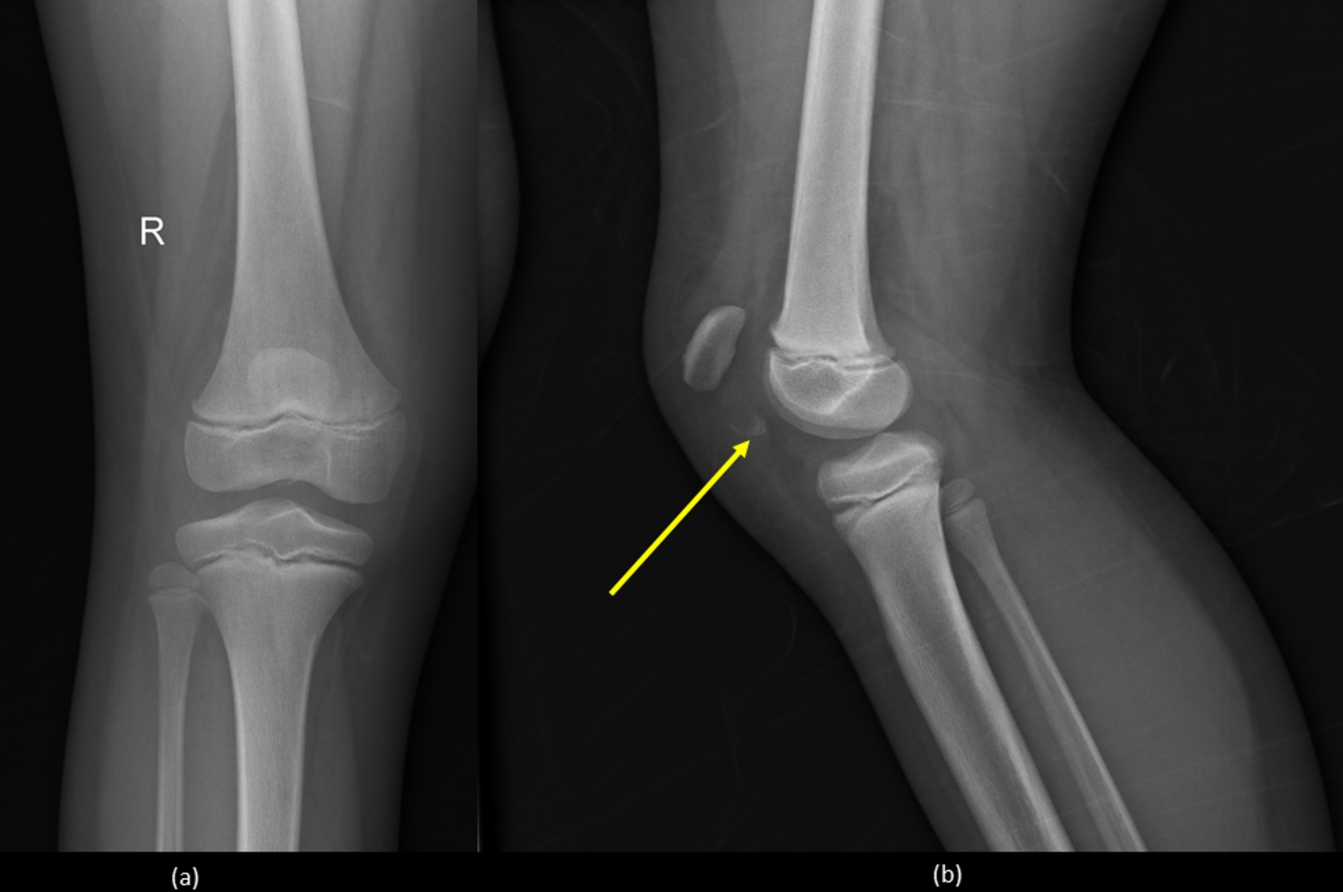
Knee radiographs. (a) Anteroposterior view and (b) lateral view showing high-riding patella with marked joint effusion, in addition to a small osteochondral fragment lying distally to the patella
